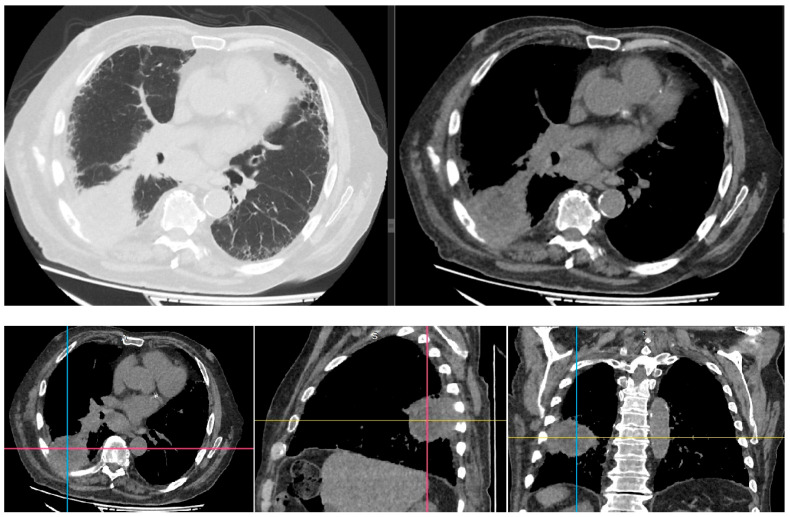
Computed tomography showing the tumor and parietal invasion.
